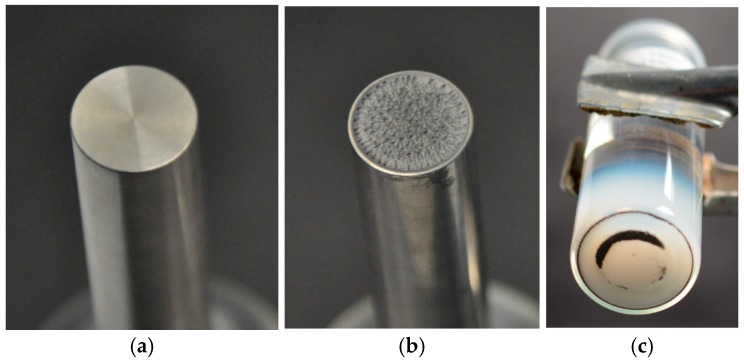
Comparison of new and used sonotrode with sonotrode abrasion as a consequence of long ultrasonic dispersion time (a,b); sonotrode abrasion sediment on the bottom of a precipitated silica suspension sample after high USD energy (c) (i.e., *E_V_*_,cal_: 1440 J/mL).
